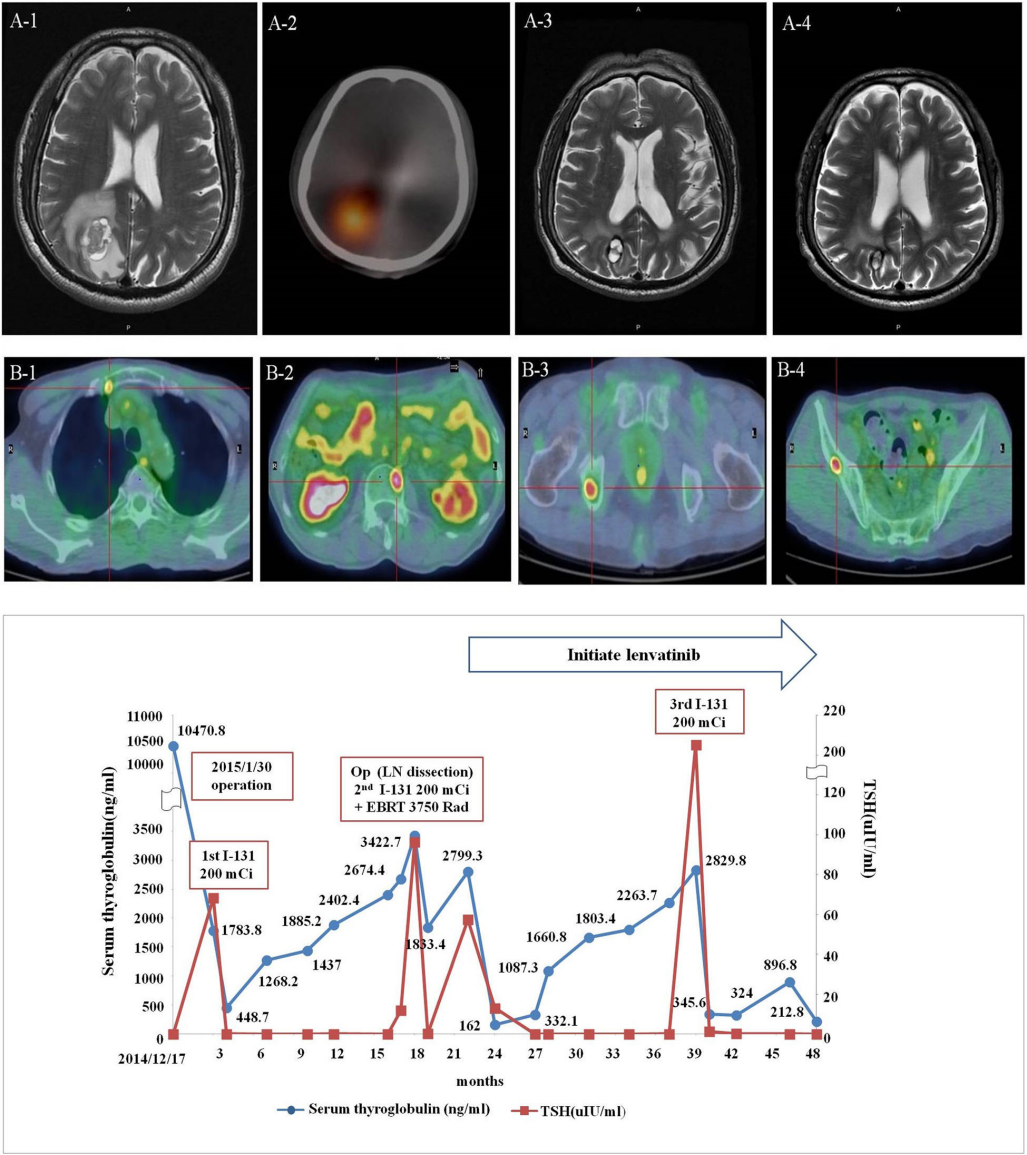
Upper panel: dynamic changes in brain and bone metastases. Hemorrhagic brain metastasis over the right occipital area was detected by brain MRI (16th month) (A-1); strong radioiodine uptake over the metastatic brain lesion was demonstrated by radioiodine whole body scan (18th month) (A-2); significant shrinkage (2.5 to 1.3 cm) of the brain metastasis after radioiodine, external beam radiation, and lenvatinib therapy, shown by brain MRI (29th month) (A-3); persistent regression of the metastatic brain lesion (39th month) (A-4). Disease progression with multiple bone metastasis was detected by 18 FDG-PET PET scan (43th month) to the right manubrium of the sternum (B-1), thoracic T4–5 spine (B-2), right ischium (B-3), and right iliac (B-4). Lower panel: the dynamic changes of serum TSH and thyroglobulin in response to various treatment modalities. The number indicates the TSH-dependent serum thyroglobulin level (ng/mL)
